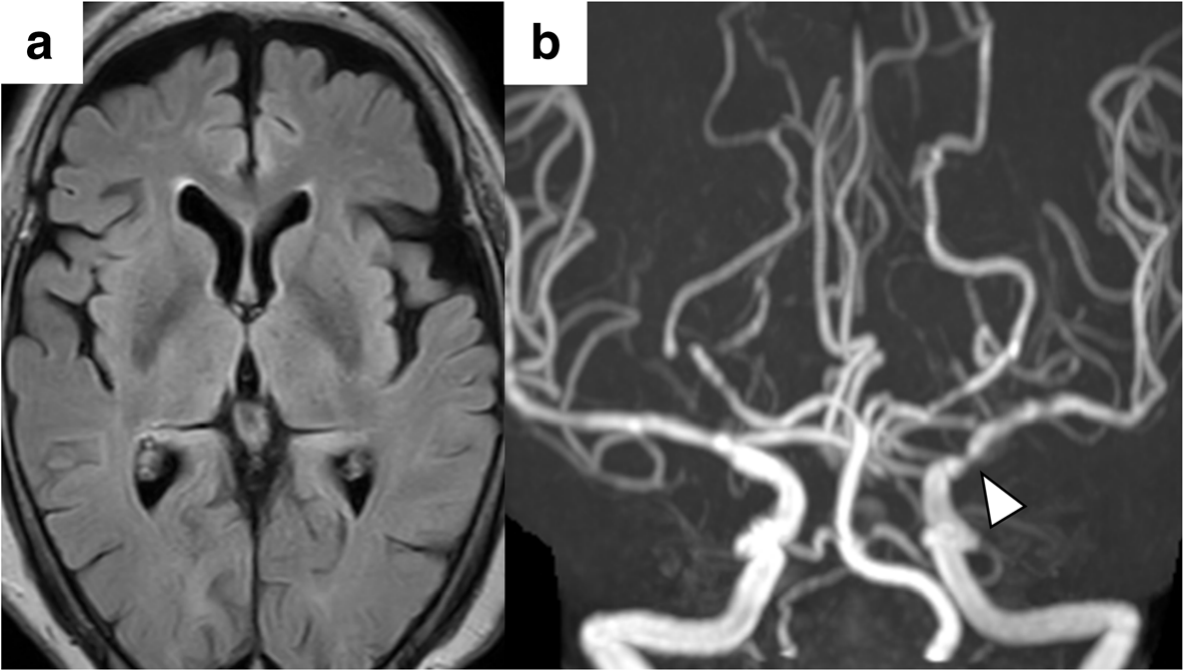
Follow-up head MRI fluid-attenuated inversion recovery image (a) and MRA (b) taken one year later showed no interval changes, including unilateral intracranial stenosis of the left internal carotid artery (b, arrowhead)
